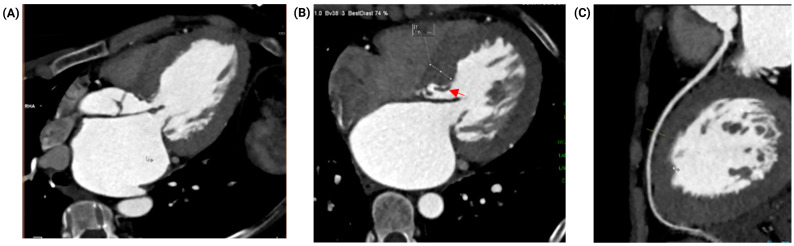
Coronary computed tomography angiography and anatomic definition of the LVOT. (A) CT findings consistent with an elongated subaortic membrane producing fixed subvalvular LVOT obstruction. (B) Marked left atrial and left ventricular dilatation with interventricular septal hypertrophy. (C) Coronary arteries without evidence of obstructive coronary artery disease. Red arrow indicates the elongated subaortic membrane.
